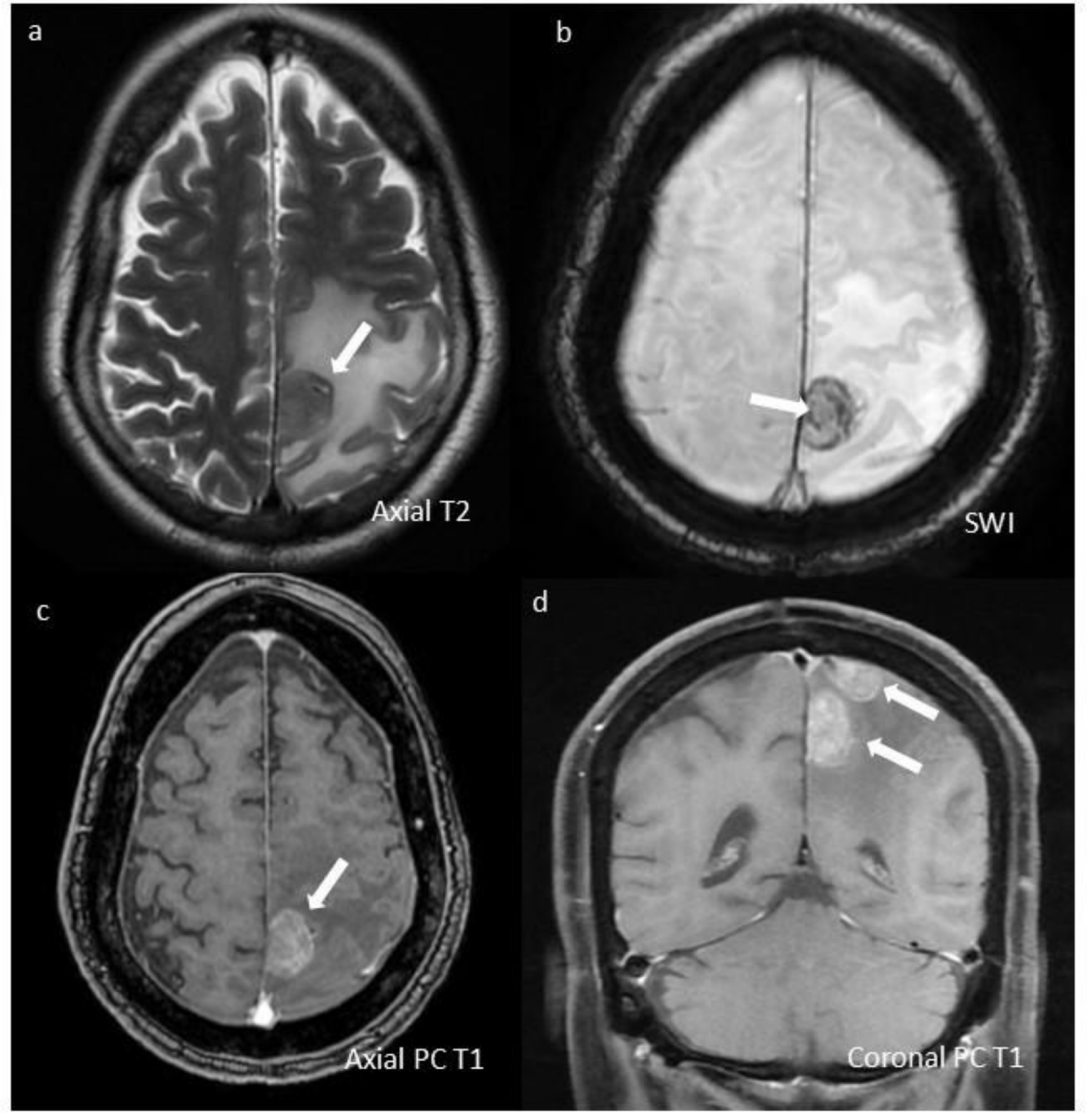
Contrast-enhanced MRI images show well-defined, extra-axial dural-based lesions (marked with white arrows) in left high parietal para-falcine region. (a) Lesion has intermediate T2 signal with significant surrounding T2 hyperintense vasogenic edema. (b) It shows blooming on SWI suggesting intralesional hemorrhage. (c,d) Two similar morphology lesions showing heterogeneous moderate enhancement are seen on post contrast T1w images
